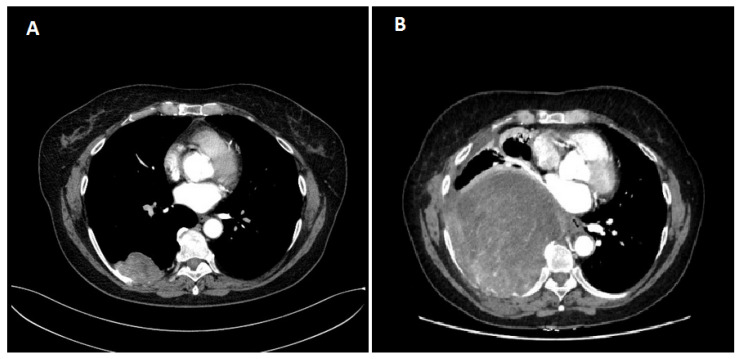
Chest CT scan. (A) first; (B) last. CT—computed tomography.
